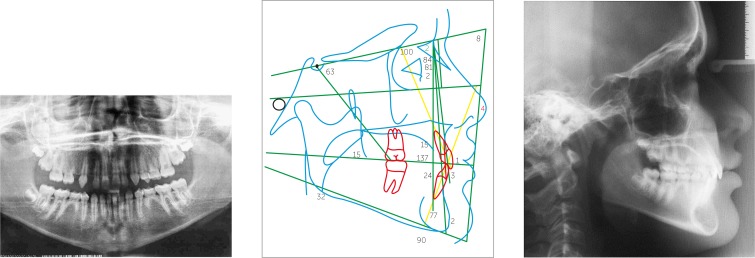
Panoramic radiograph confirms hypodontia of teeth #14, 15, 25, 35 and 45.
